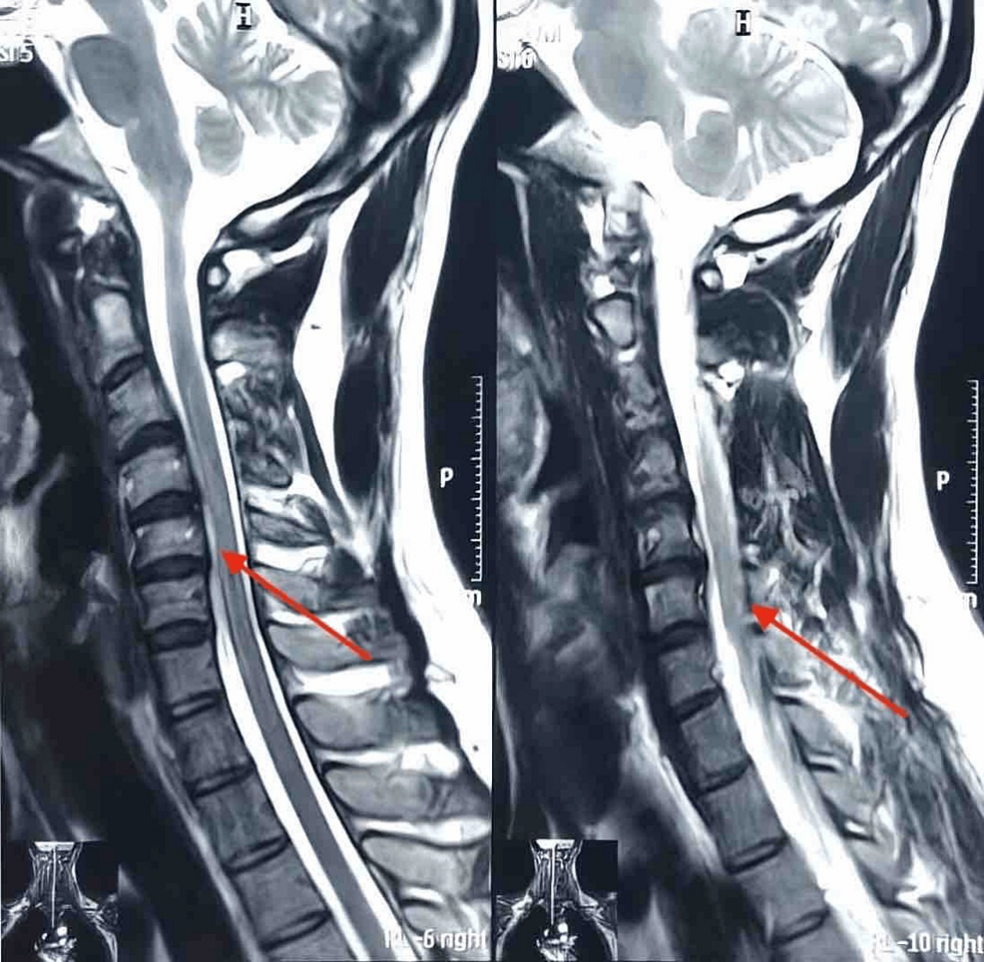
T2 MRI sequence showing cervical myelomalacia
